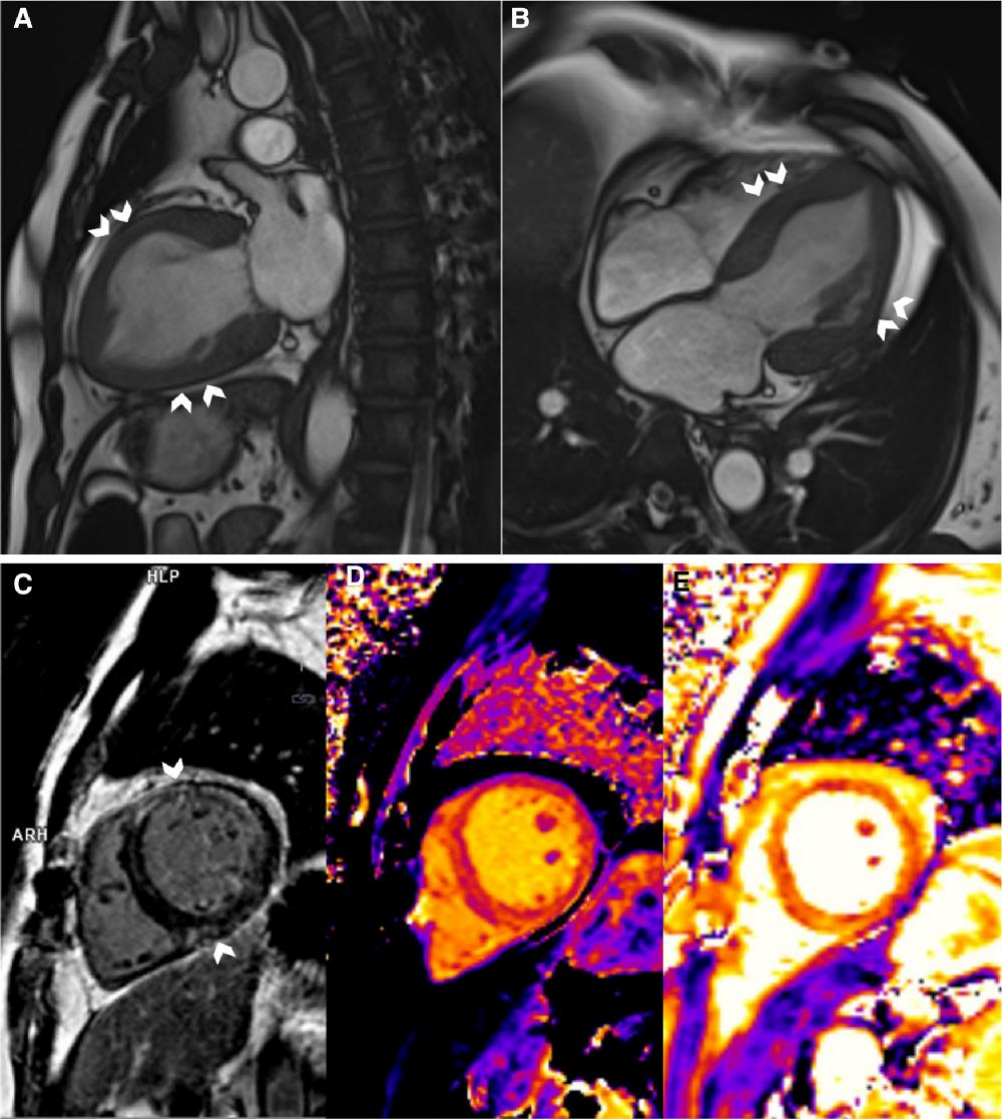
Cardiac magnetic resonance imaging. (*A* and *B*) Systolic frames of cine images showing akinesia of the mid-segments, i.e. mid-anterior, septal, lateral, and inferior (chevron arrows), and normokinesia of the basal and apical segments. (*C*) Mid-wall Late Gadolinium enhancement at the mid-anterior, anteroseptal, anterolateral, and inferior (chevron arrows) segments. (*D* and *E*) Parametric mapping of the corresponding slice, showing increased global native T1 (*D*) and T2 (*E*) relaxation times, implying the presence of oedema.
